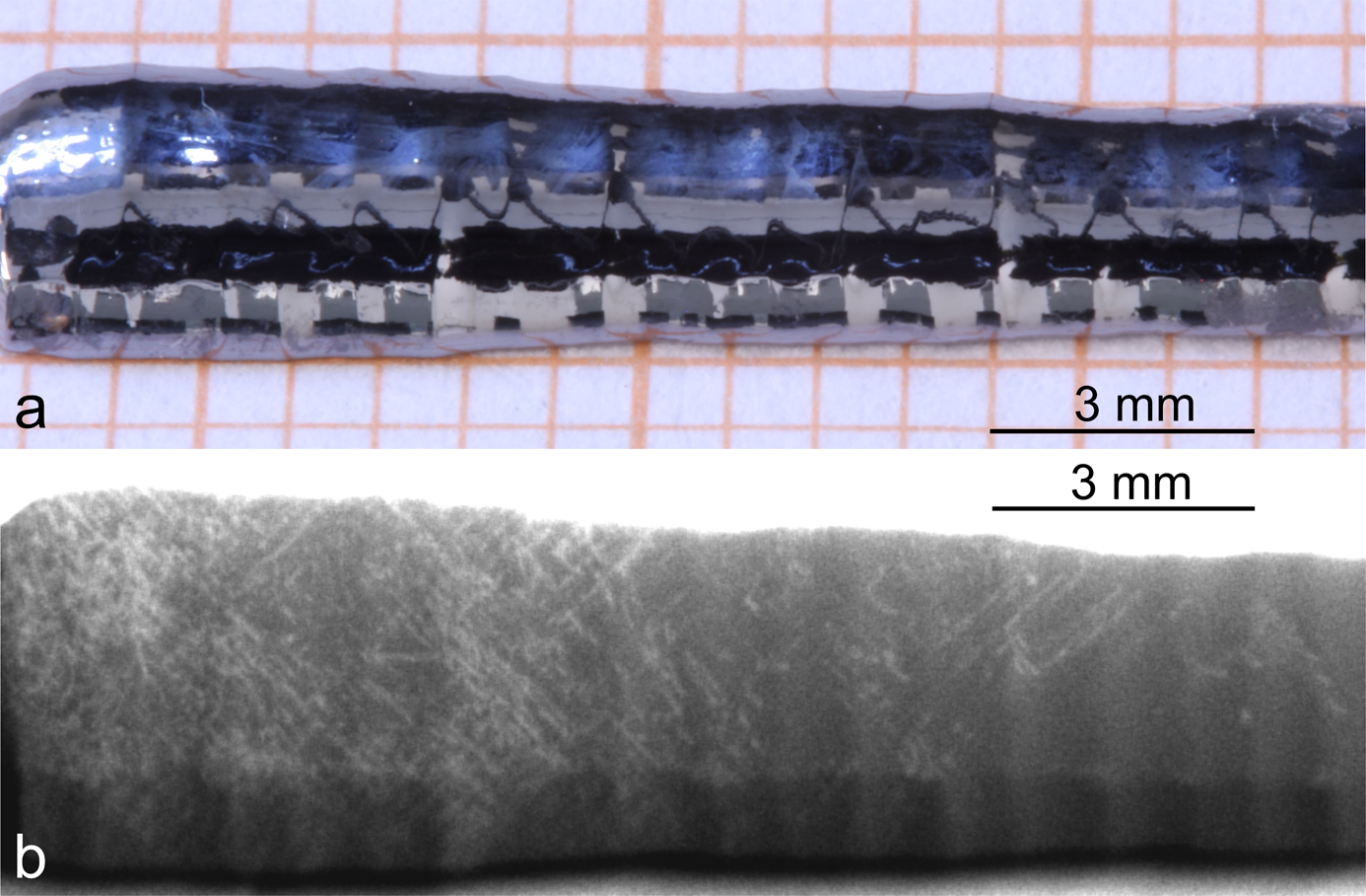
(*a*) A photograph of the investigated region of sample Si-3 with a similar viewing direction to the X-ray beam. (*b*) A superimposed greyscale-coded 2D intensity plot of the peak flanks and peak maxima of the 337 and 448 reflections of the sample shown in panel (*a*) with a slight increase in contrast (Rh source with AMS).
